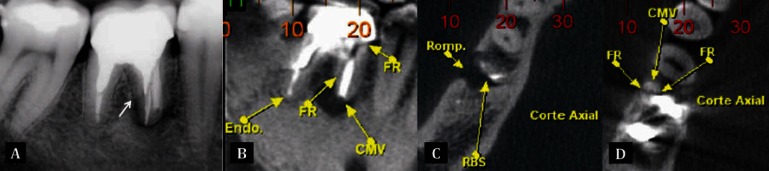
A) Radiograph of tooth 46 showing a possible line of fracture (white arrow); B,C,D) CBCT images in the sagital and axial planes of the tooth clearly shows fracture line (FR) and resorption area (RBS)
